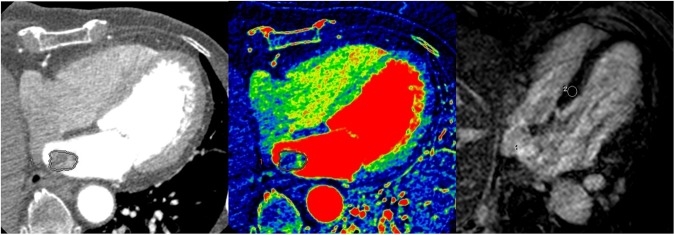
A 72-year-old female patient diagnosed with a myxoma. (A) On enhanced CT, a 2.4 × 1.5-cm well-defined mass was noted in the left atrium. The volume of the mass was 3.37 cm^3^ and the CT attenuation value of the intra-cardiac mass was 40.00 HU. (B) On the iodine (water) image, the iodine concentration of the mass within the VOI was 5.04 mg/ml. (C) On LGE image, the mass shows strong contrast enhancement; the SI ratio of the mass was 20.50.
